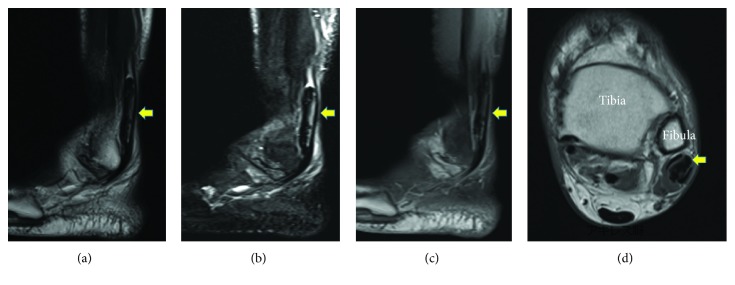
Sagittal T1- and T2-weighted MR images showed an elliptical mass of low intensity partially with high intensity with no contrast effect. Axial T1-weighted MR images showed a low-intensity mass (yellow arrow) in the peroneal tendon sheath, which seemed to compress both the peroneal brevis and longus tendons. (a) Sagittal T1-weighted image. (b) Sagittal T2-weighted image. (c) Sagittal enhanced image. (d) Axial T1-weighted image.
